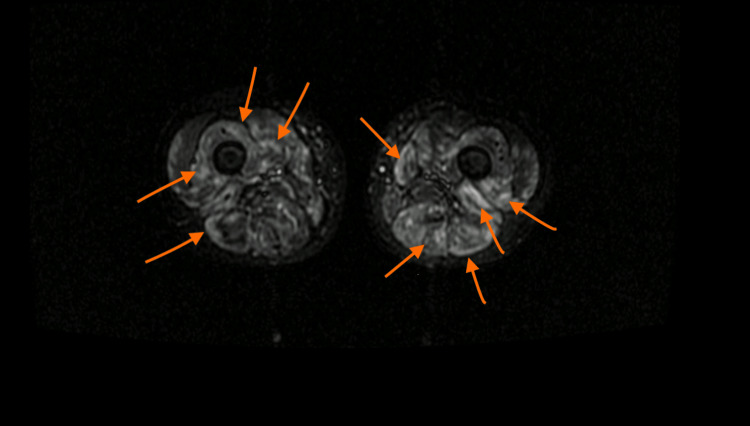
Axial STIR MRI image through patient thighs revealing heterogeneous hyperintense signal (orange arrows) affecting all the compartments STIR - short TI inversion recovery
